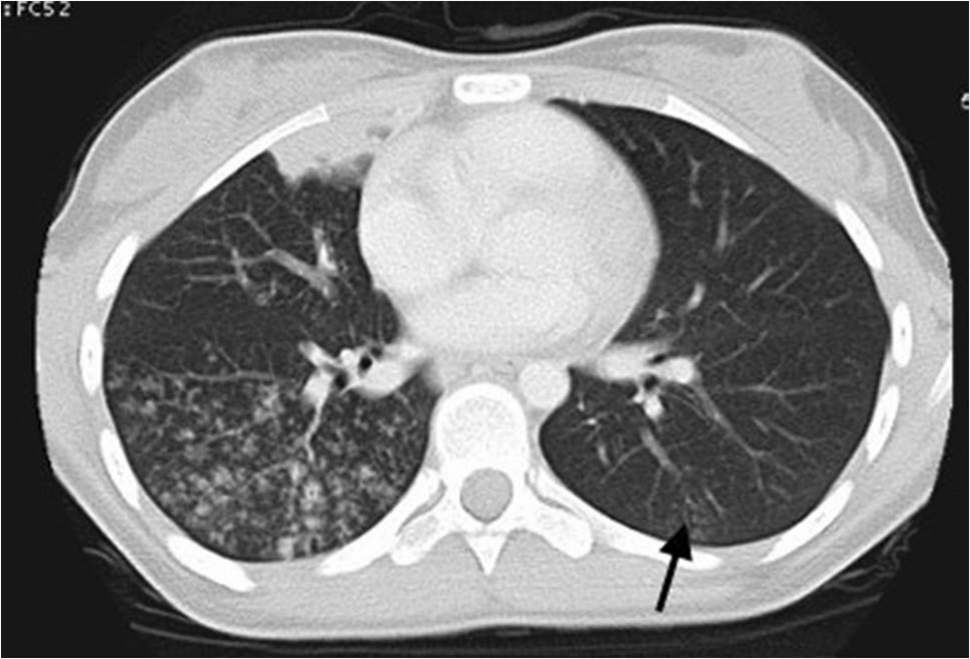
A 12-year-old girl with pulmonary tuberculosis. Axial computed tomography image (lung window) demonstrates multiple coalescing centrilobular nodules in the right lung. A small patch of consolidation is also seen. A few ill-defined centrilobular nodules are also seen in the left lung (*arrow*)
